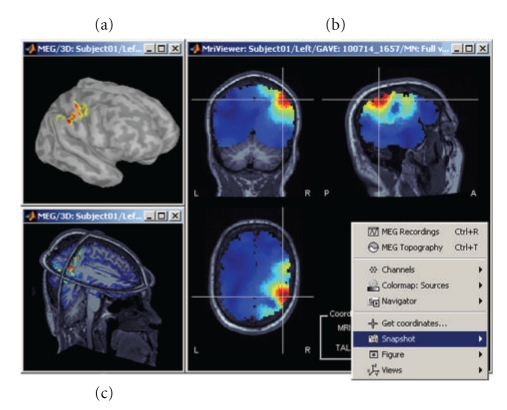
A variety of options for the visualization of estimated sources. (a) 3D rendering of the cortical surface, with control of surface smoothing; (c) 3D orthogonal planes of the MRI volumes; (b) conventional orthogonal views of the MRI volume with overlay of the MEG/EEG source density.
